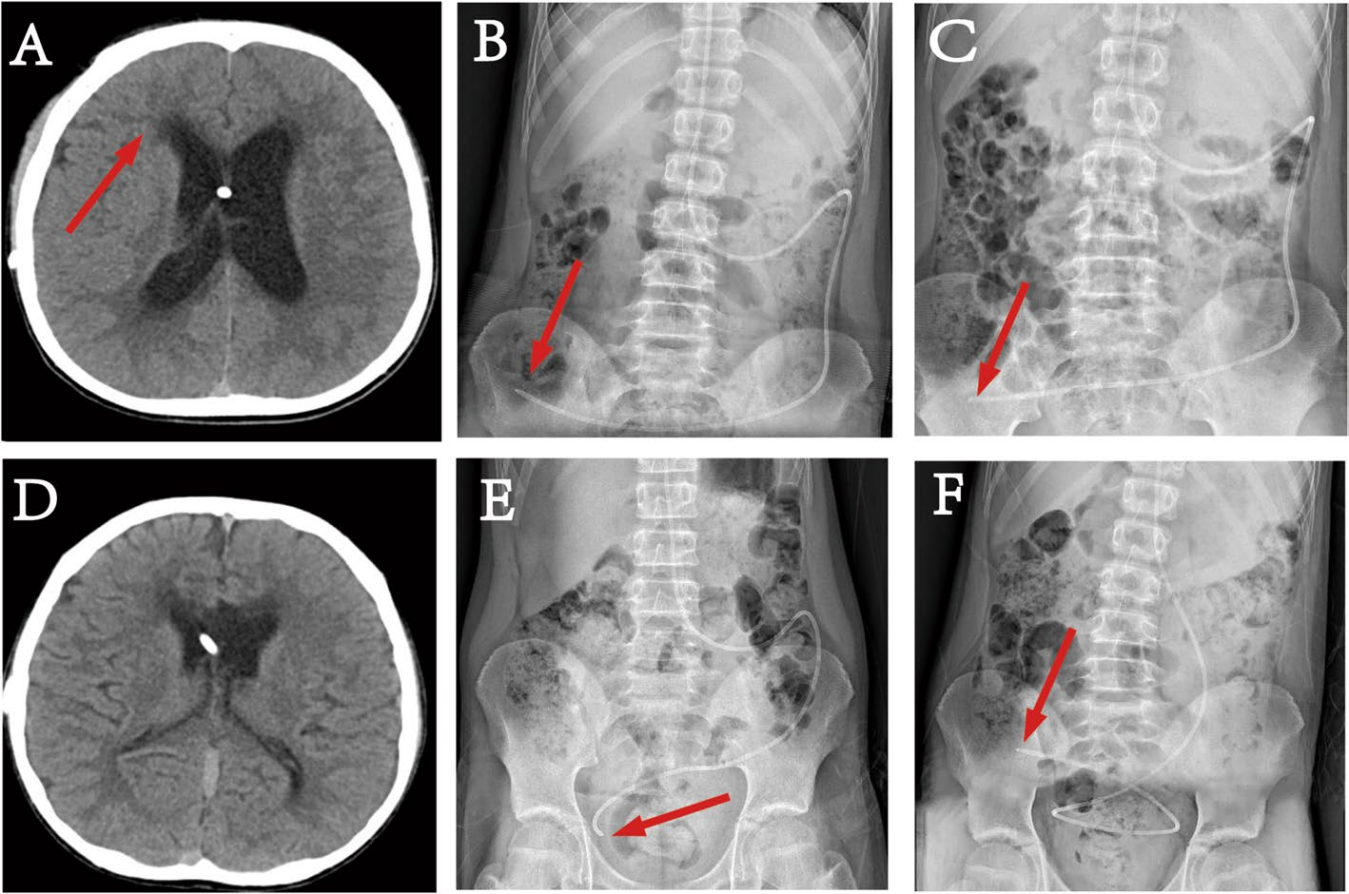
Case presentation before and after shunt adjustment of the abdominal segment of the shunt in patients with shunt insufficiency due to abdominal segment factors after ventriculoperitoneal shunt. A–F Male, 11 years old: Right lateral ventriculoperitoneal shunt was performed due to “three-ventriculoposterior space-occupying lesions with hydrocephalus.” Eight years after the first surgery, he complained of “intermittent headache for 1 month, aggravated with vomiting for 5 days.” A CT before the adjustment of the abdominal end of the shunt catheter after VP showed bilateral dilatation of the lateral ventricle, interstitial edema of the frontal angle of the lateral ventricle (red arrow), and swelling of brain tissue. B The first anteroposeural X-ray of the abdomen before adjustment of the shunt abdominal end shows the position of the end of the shunt abdominal end (red arrow). C The second anteroposeural X-ray of the abdomen before the adjustment of the abdominal end of the shunt (reviewed 5 h after the first X-ray) showed that the position of the distal end of the abdominal end of the shunt was fixed and unchanged (red arrow). D CT after the adjustment of the abdominal end of shunt catheter showed that bilateral lateral ventricles were smaller than before, interstitial brain edema was reduced, and brain tissue swelling was reduced. E The position of the end of the abdominal end of the shunt catheter (red arrow) was shown in the first anteropoetal abdominal X-ray after the adjustment of the abdominal end of the shunt catheter. F The second anteropoetal X-ray of the abdomen after the adjustment of the abdominal end of the shunt shows the change in the position of the abdominal end of the shunt (red arrow)
